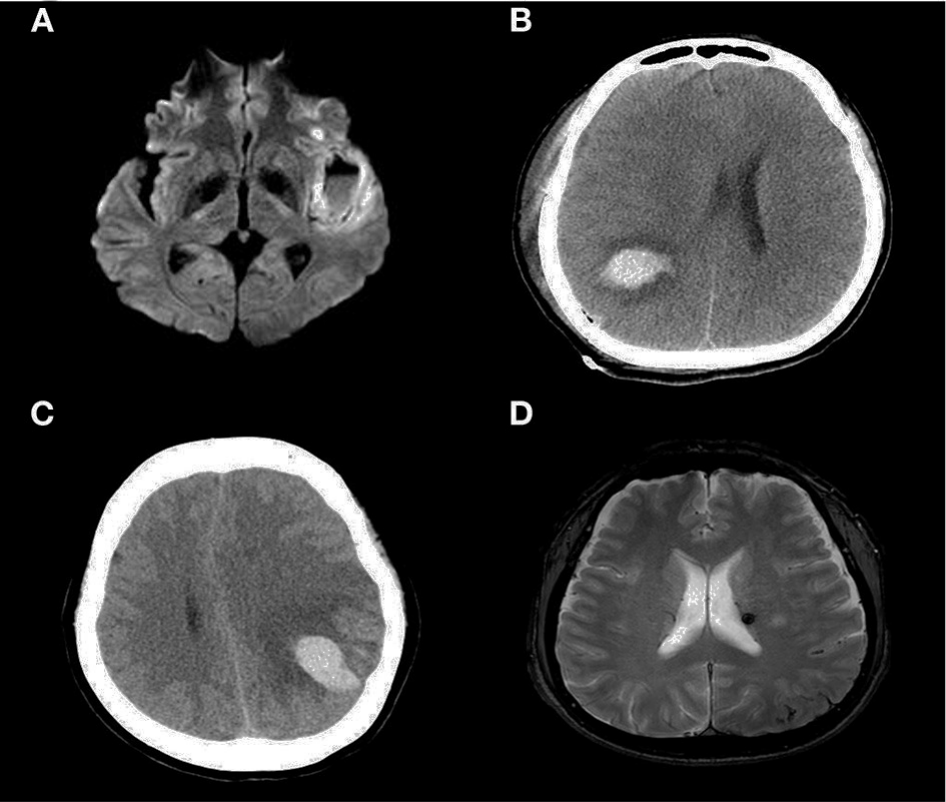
Diffusion-weighted image showing a large, left-side, insular cortical hemorrhage with a distinct fluid level (A). A CT scan showing a parenchymal hemorrhage in the right temporal-parietal area (B) and left parietal cortex (C). A newly developed (small) subarachnoid hemorrhage in the left parietal convexity and microbleeds in the thalamus (D).
